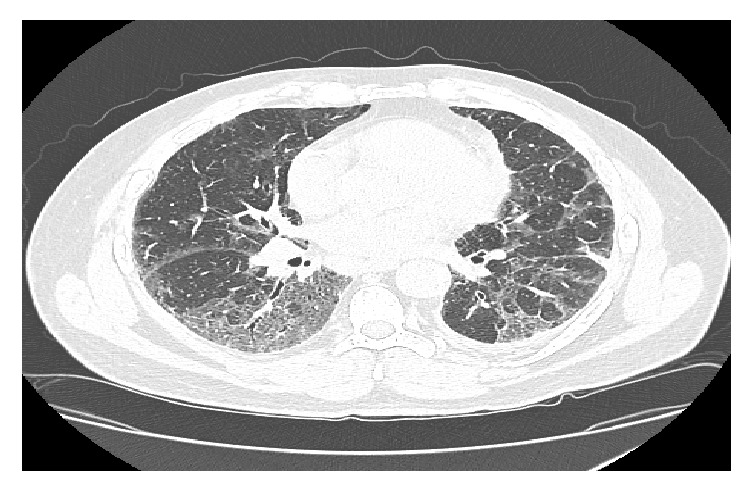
CT-high resolution chest without contrast. Taken 15 months before acute exacerbation. Ground glass opacities with thickening of the interlobular septa, predominantly in the lung bases.
